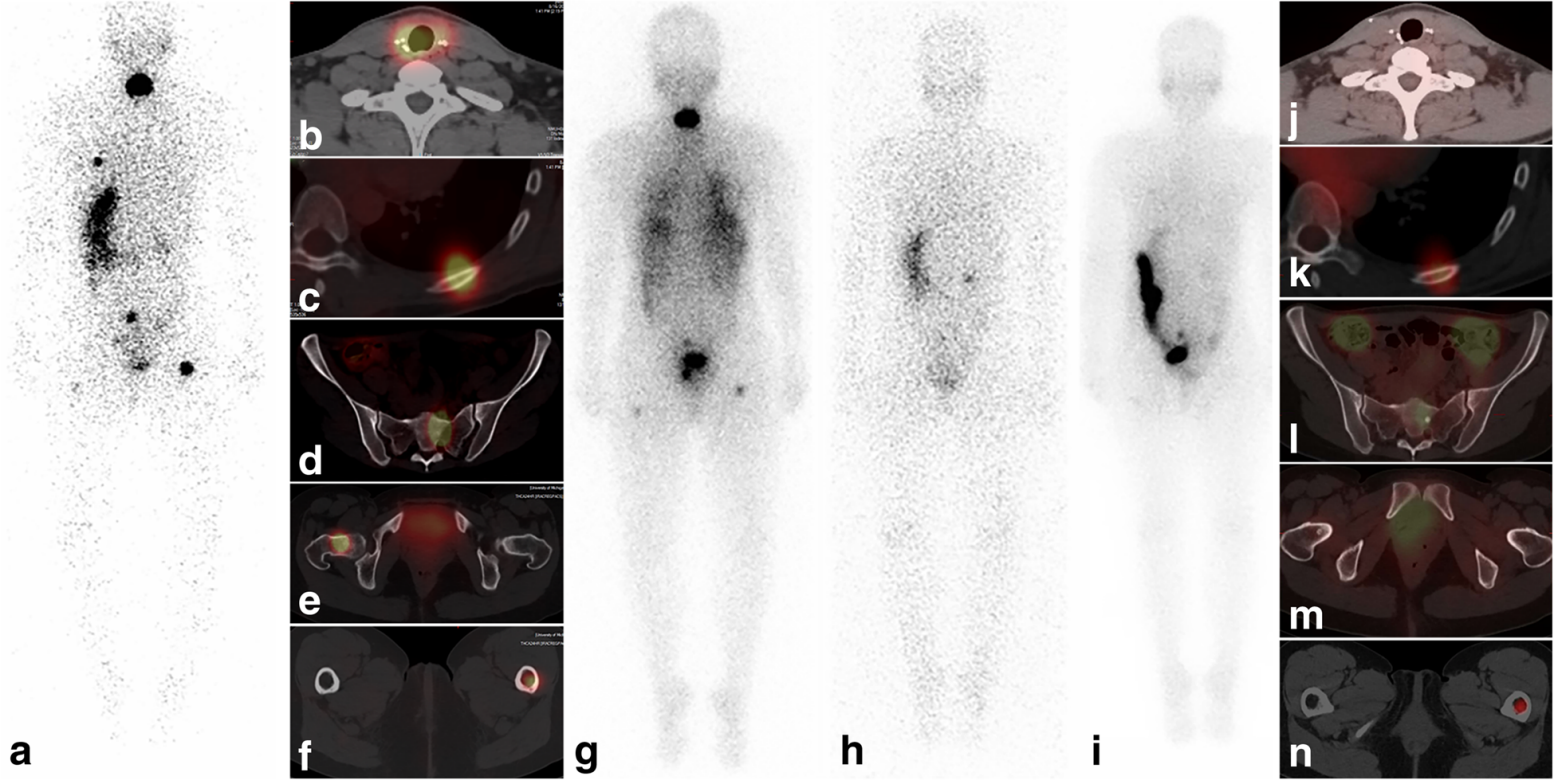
Nuclear medicine imaging at initial evaluation and subsequent follow-up of metastatic struma ovarii. Diagnostic 131-I planar scan, posterior view (a) demonstrates central neck activity and abnormal activity in the left hemithorax, central pelvis and bilateral proximal femurs. Diagnostic SPECT/CT demonstrates thyroid remnant tissue in the thyroidectomy bed (b) and skeletal metastatic disease localized to left 9th rib (c), sacrum (d) and bilateral proximal femurs (e, f). Post-therapy 131-I planar scan, posterior view (g) demonstrates diffuse lung activity consistent with miliary pulmonary metastases in addition to focal central neck activity and skeletal metastatic foci. Follow-up diagnostic planar 131-I scan, posterior view (h) demonstrates physiologic radiotracer activity in the stomach, and urinary activity in the right renal collecting system and bladder, without foci of abnormal activity. Post-therapy 131-I scan, posterior view (i) obtained after 100 mCi therapeutic challenge ascertained resolution of pulmonary miliary metastatic disease, but residual foci of activity were demonstrated in the left hemithorax and pelvis and confirmed on SPECT/CT in the left ninth rib (k), sacrum (l), and left proximal femur (n) consistent with a partial therapeutic response in the skeleton. Focal sclerosis without focal 131-I uptake is demonstrated in the right femoral neck, consistent with treatment response and bone healing (m). Complete therapeutic response is demonstrated in the thyroidectomy bed (j)
